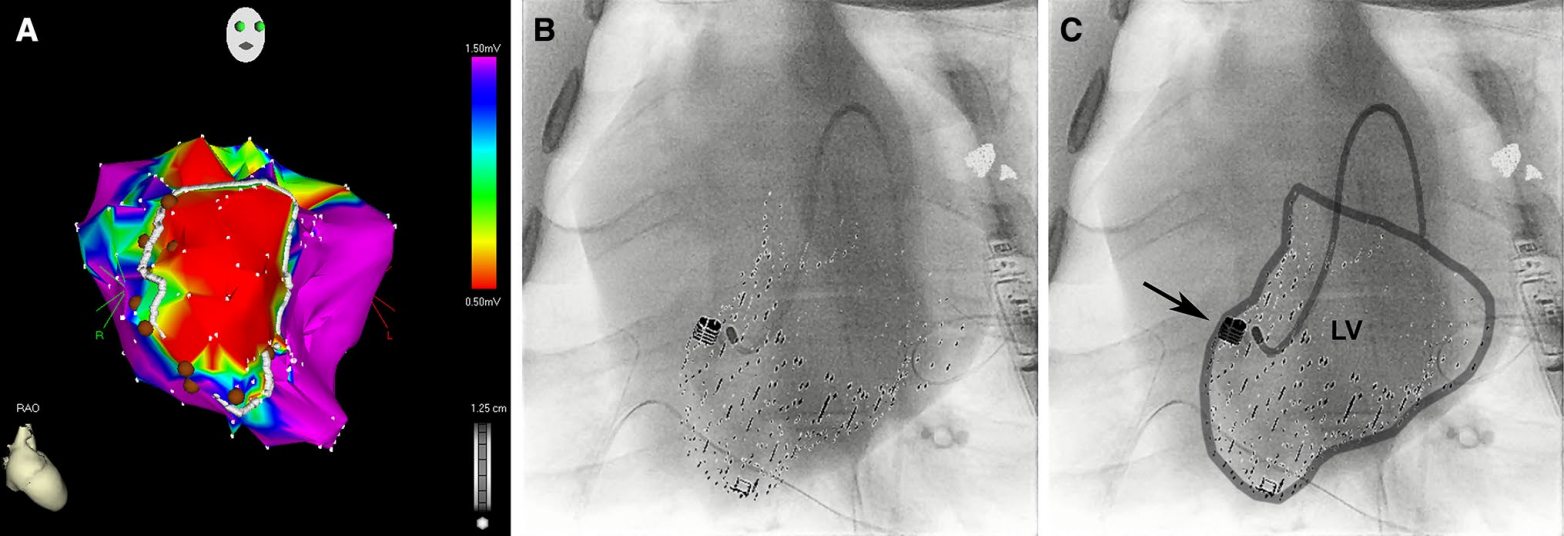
Examples of NOGA and CARTBox injection procedure. a Example of a NOGA bipolar voltage map (color scale: 0.5–1.5 mV) in right anterior oblique view, with design line (white) to indicate the infarct border zone. The injections are shown as brown circles. b Anterior-posterior X-ray fluoroscopy image fused with the CARTBox2 modified MRI scan. The left ventricular endocardium (small dots) and the injection target (large dot). c The same image as shown in b, the endocardial wall and catheter are visually enhanced. The arrow indicates the injection target defined on late gadolinium enhanced MRI
